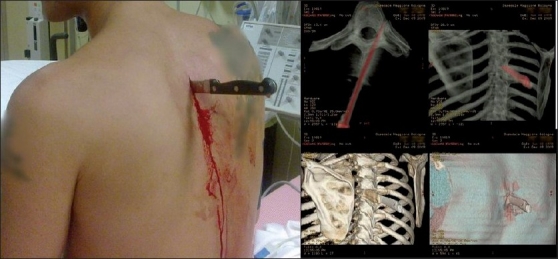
Clinical presentation and CT 3D reconstruction
